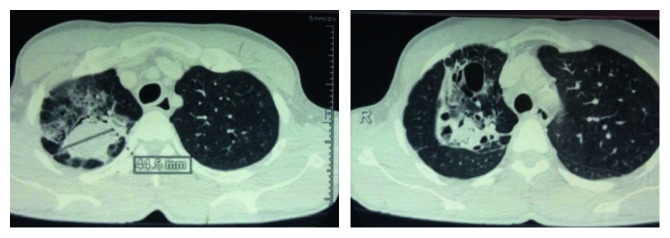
Computed tomography of the chest. A transverse section showing a right-sided complex lesion of the upper lobe adherent to the chest wall.
